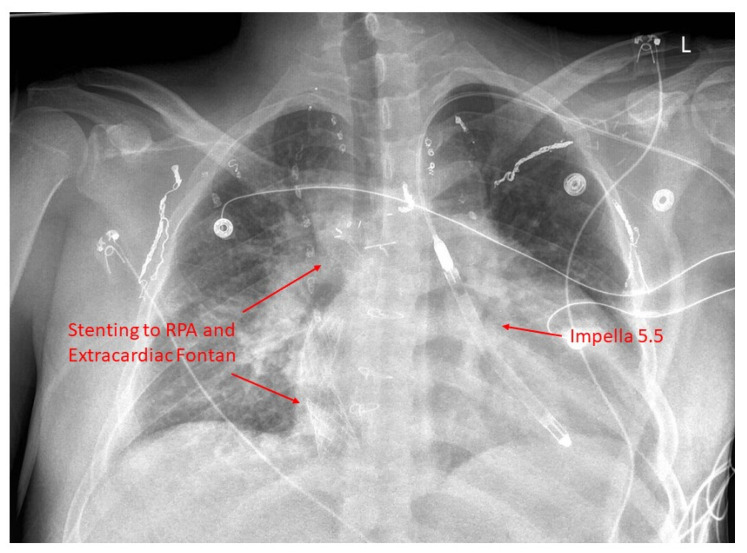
Chest X-ray of a patient with hypoplastic left heart and extracardiac Fontan with temporary mechanical circulatory support as bridge to heart transplant.
